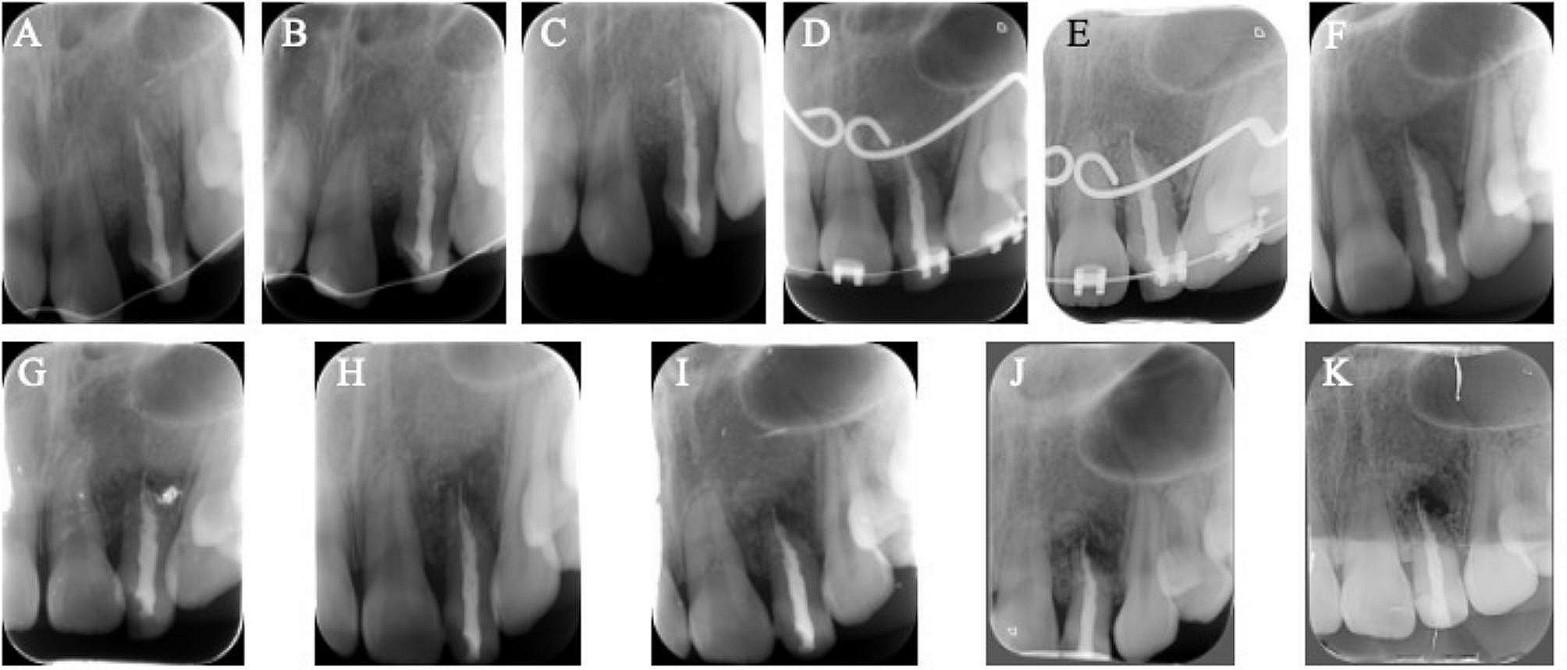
Follow-up periapical radiographs of Case 1 with a fused left lateral incisor. (A–K) periapical radiographs of 1, 2, 3, 6, 12, 18, 24 months, and 3, 4, 5, and 11 years after replantation, respectively
